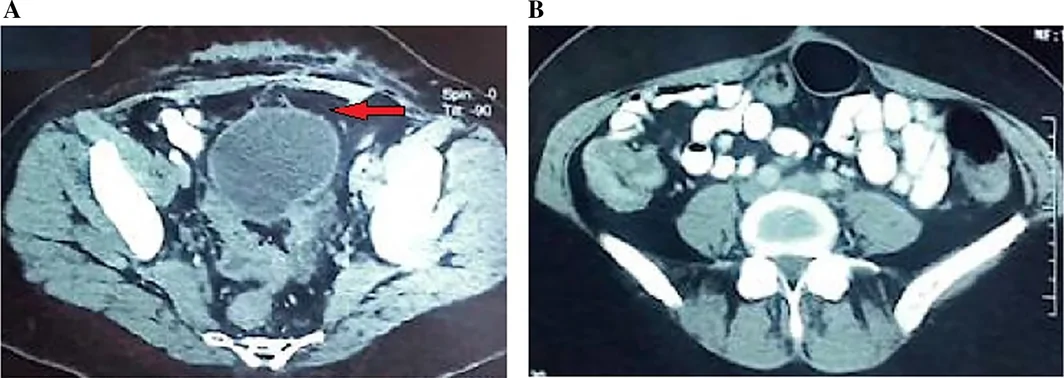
Contrast‐enhanced computed tomography (CT) images obtained before and after olaparib treatment. (A) Baseline CT scan showing the recurrent pelvic lesion (arrow) before initiation of olaparib. (B) Follow‐up CT scan demonstrating a significant reduction in lesion size after treatment.
